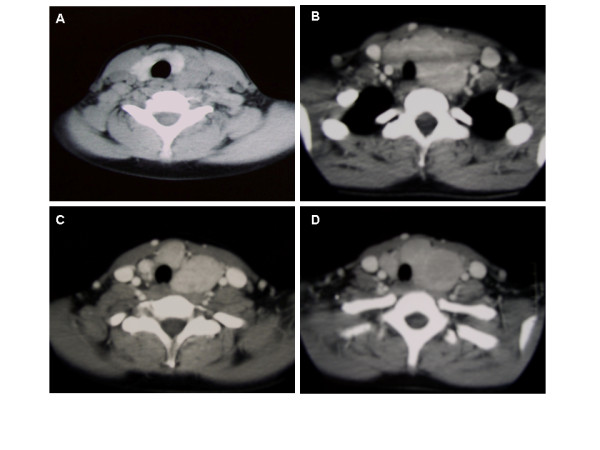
CT scan showed that the neck mass, a multiple lymph node enlargement, with an high contrast-enhancement, originated in the anterior-superior mediastinum and cause a serious right dislocation of the trachea (A-D).
